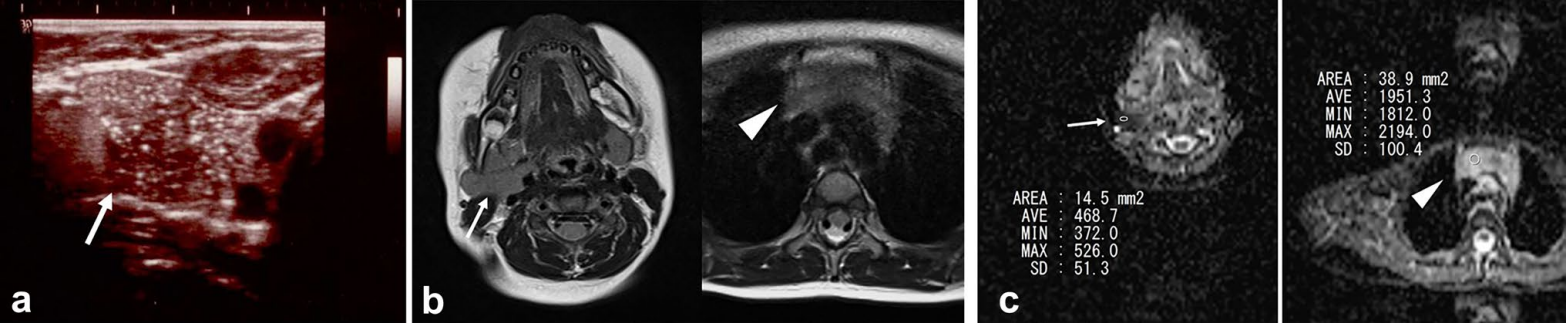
Two-year-old girl with ectopic thymus. a Ultrasonography revealed mass (arrow) at the right parapharyngeal space. The mass has scattered dots and lines of high echoic areas, which are known as “starry sky” or “dot and dash” appearance. b On T2 weighted image, mass (arrow) shows slightly high signal intensity which are same as that of normal mediastinal thymus (arrow head). c Apparent diffusion coefficient (ADC) value of mass (arrow: 468.7 x 10^−6^mm^2^/sec) was lower than that of normal mediastinal thymus (arrow head: 1951.3 x 10^−6^mm^2^/sec)
